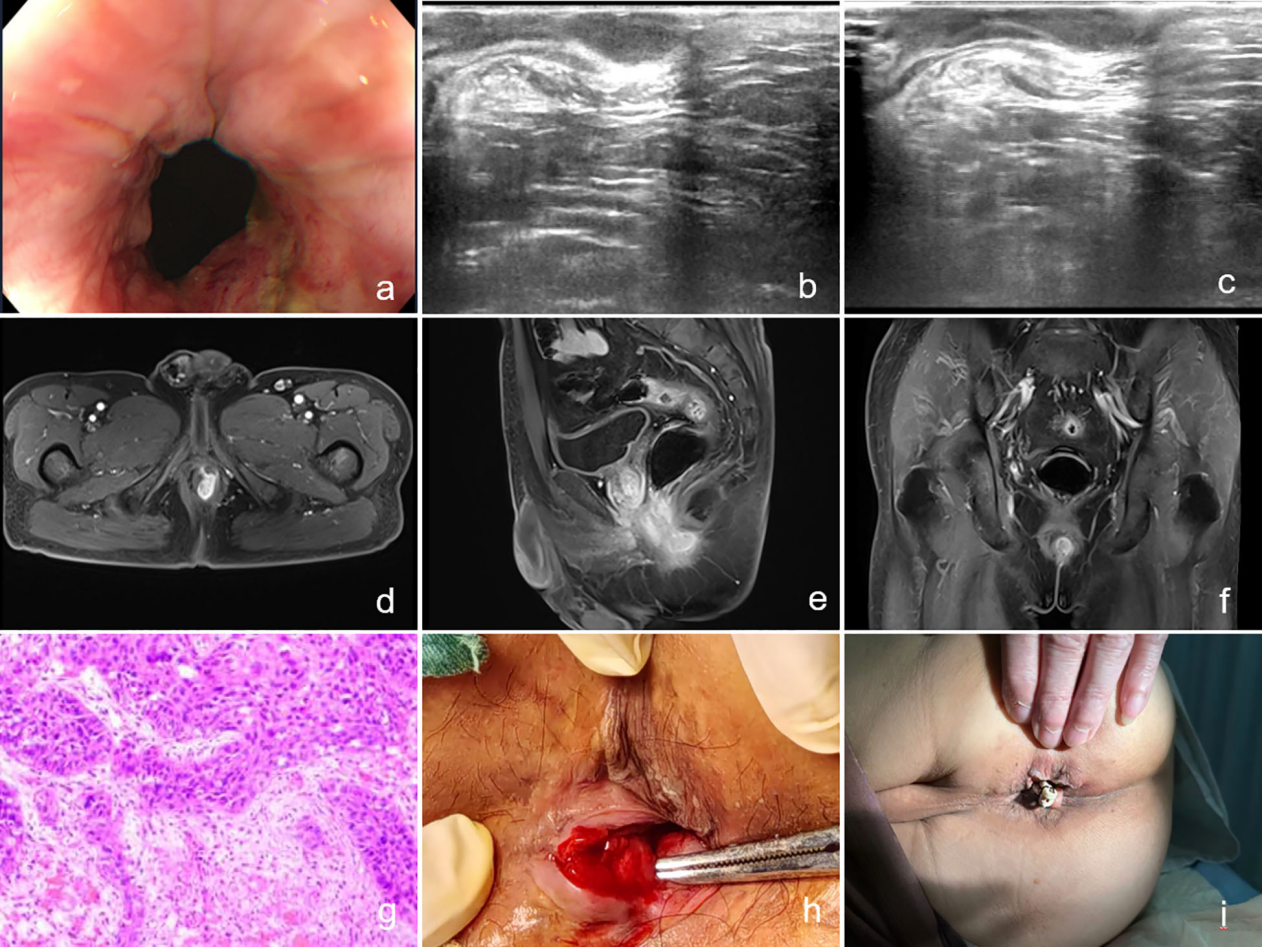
(a) Image of the anal orifice taken by colonoscopy; (b, c) Images from two-dimensional endorectal ultrasound; (d–f) Magnetic resonance images; (g) Postoperative pathology; (h) Image on the day of surgery; (i) Image on the second postoperative day.
